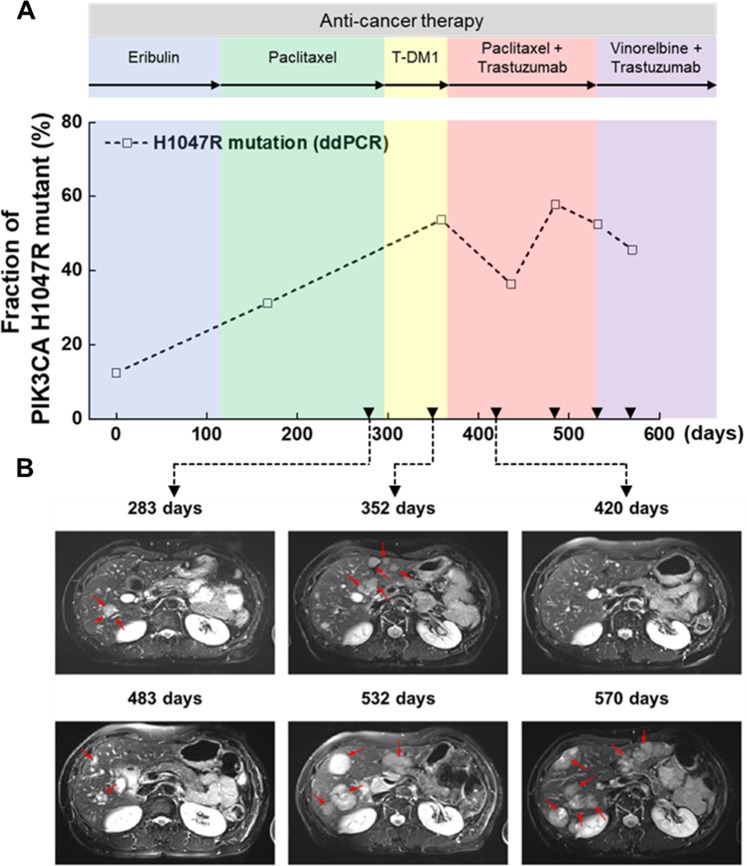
Clinical validation of PIBEX chip in liquid biopsy of breast cancer. The *PIK3CA* mutation in *HER-2* type of breast cancer was monitored in liver metastasis using DNA extracted from the PIBEX chip. a Fraction change of *PIK3CA* H1047R mutation in ctDNA was detected by ddPCR. Each cancer therapy image is labeled with the background color of the graph. b Hepatic metastasis from breast cancer in abdominal MRI images. Red arrows indicate metastasis of breast cancer in liver. Inverted triangles of a represent the matching MRI images of b.
